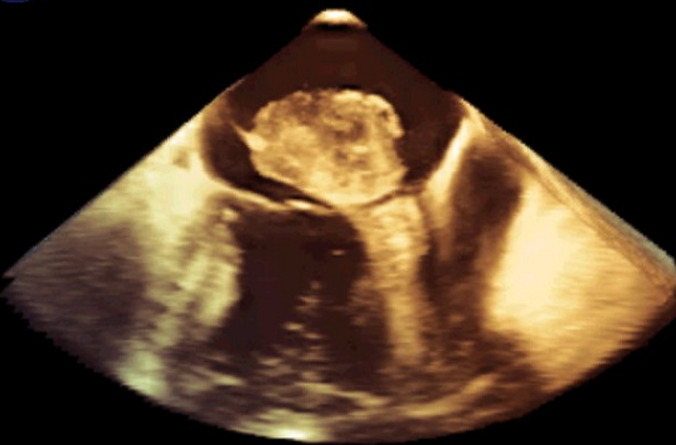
left atrial mass on 3D transoesophageal echocardiographraphy
